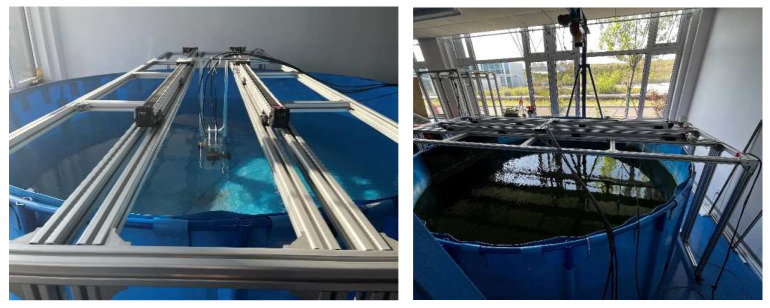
Error calibration platform for the transverse ultrasonic transducer.
